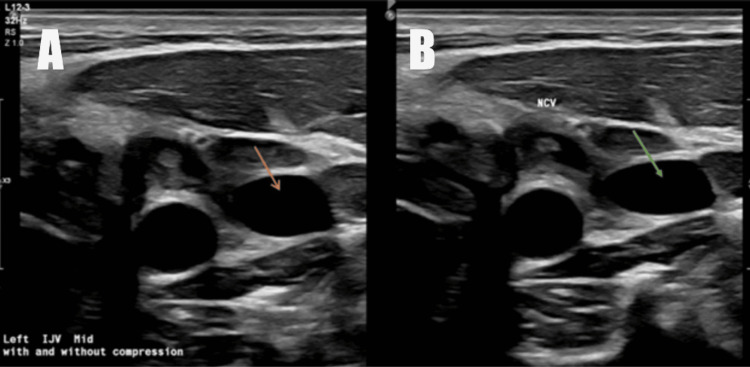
Ultrasound of the left internal jugular vein showing a clot (arrows) without compression (A), which persists with compression (B).
